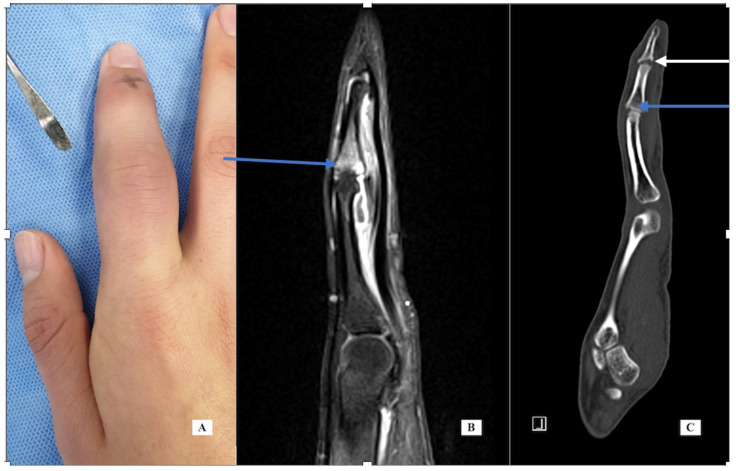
Case 1—clinical photograph of the enlarged PIP joint (A); MRI of the finger, sagittal view (B) demonstrating bone marrow edema at the base of P2; CT scan of the finger (C) demonstrating central erosions at the DIP joint (white arrow) and dorsal erosion at the PIP joint (blue arrow).
